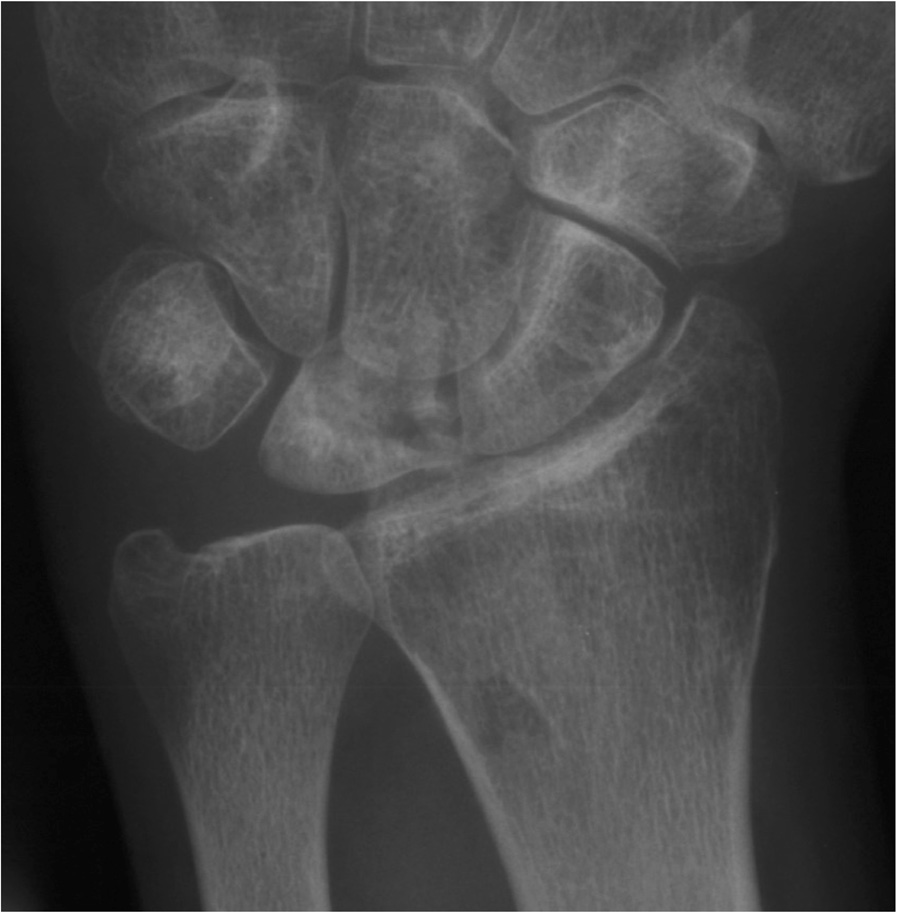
Post-operative x-rays of the left wrist of of a 44-year-old male patient who had undergone VBG
